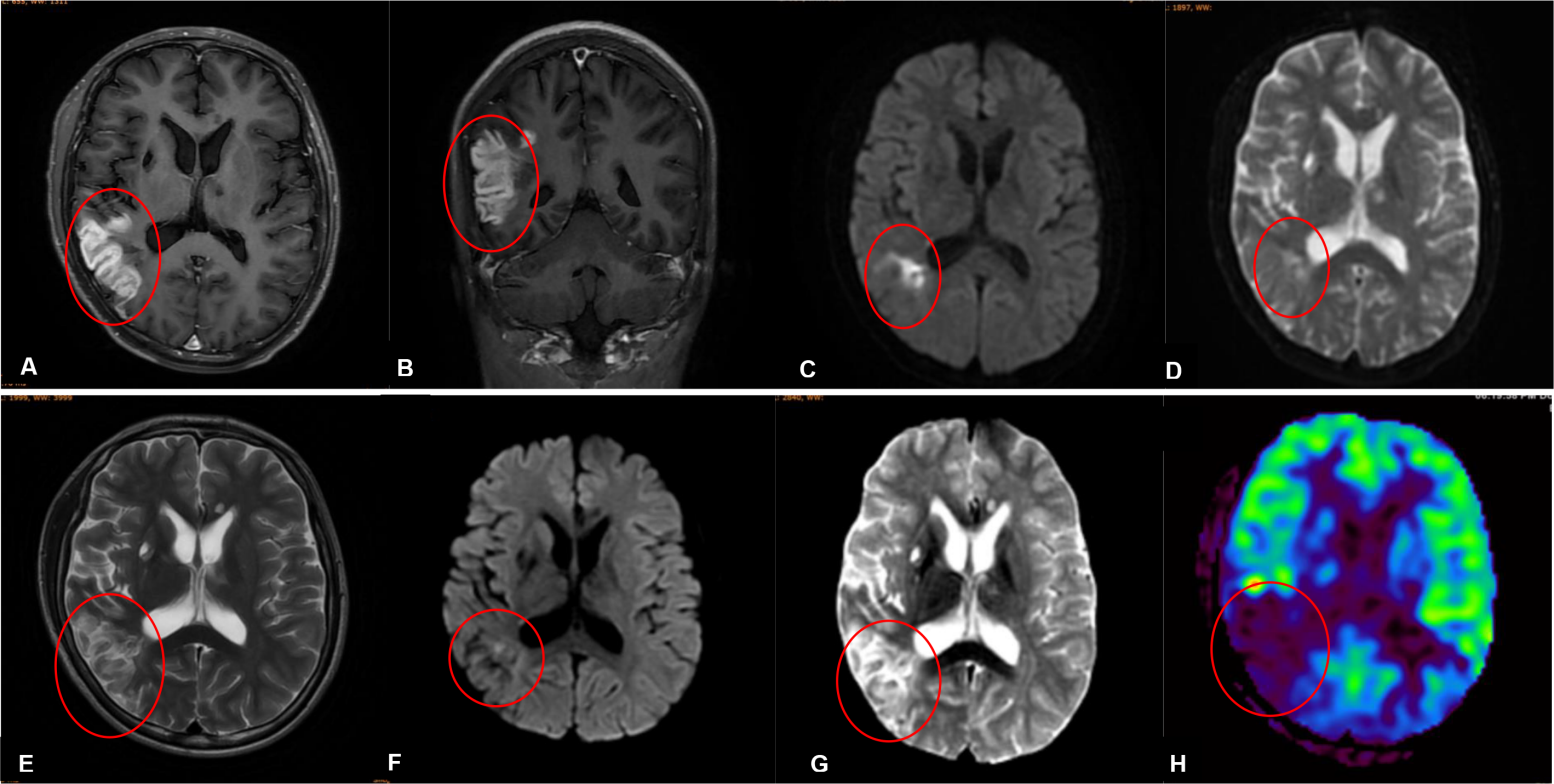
(A-D) Imaging findings obtained prior to his second admission. (E-H) Imaging findings obtained on the third day of his second admission. (A, B) Contrast-enhanced magnetic resonance imaging scans revealed patchy abnormal signals in the right temporal and parietal lobes with significant gyri-like enhancement. (C) The DWI in the right temporal and parietal lobes presented high intensities with significant limited diffusion. (D) MRI findings on ADC image in the right temporal and parietal lobes presented low intensities. (E) MRI findings on the third day of his second admission revealed lessons with high intensities on T2-weighted images in the right temporal and parietal lobes. (F) MRI findings on DWI image in the right temporal and parietal lobes presented slightly high intensities with limited diffusion. (G) MRI findings on ADC image in the right temporal and parietal lobes presented high intensities. (H) Arterial spin labeling (ASL) showed that cerebral blood perfusion in the right temporal, occipital and parietal lobes decreased significant.
